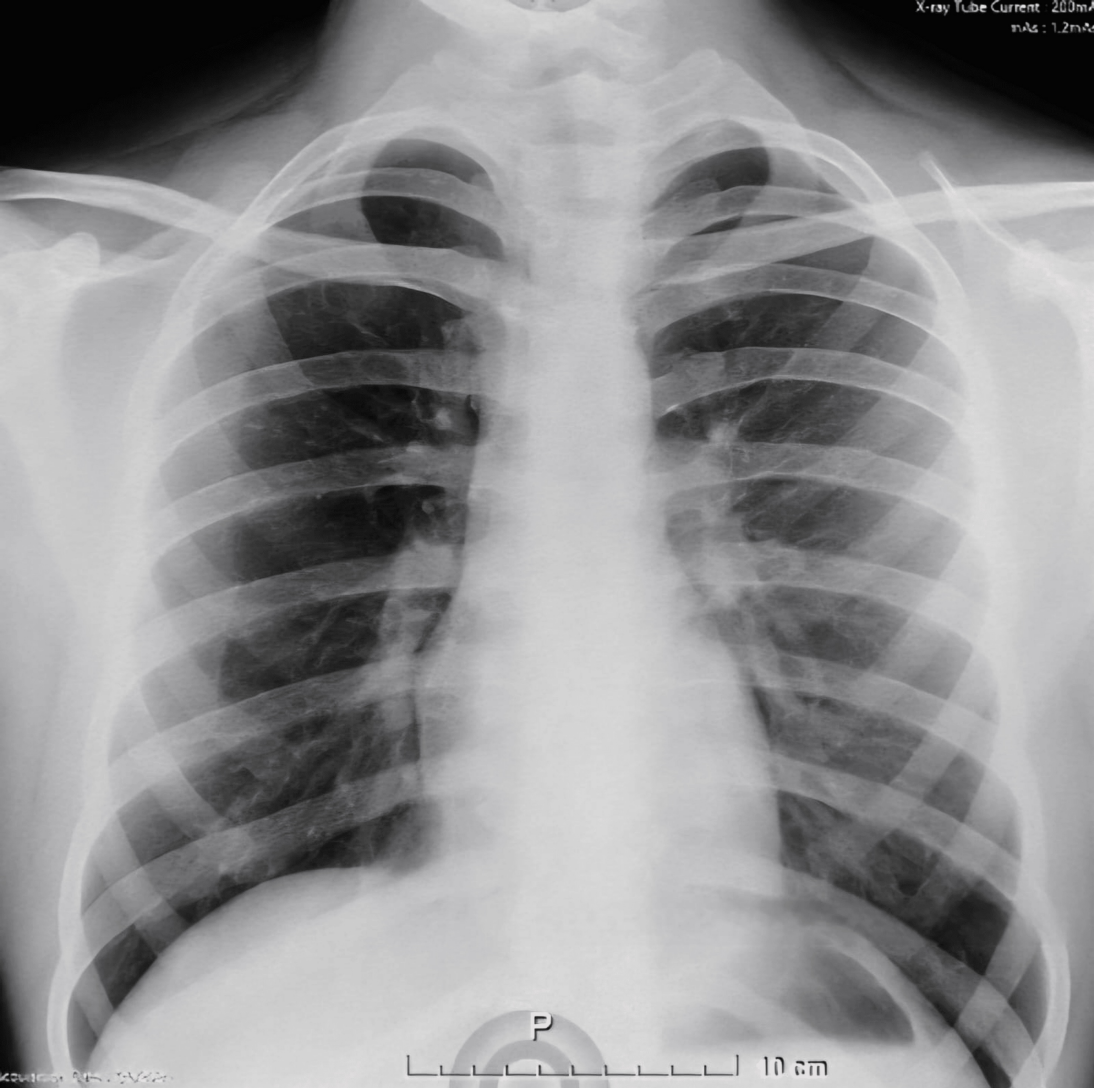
Chest X-ray The lungs are well aerated with no evidence of infiltrates, consolidations, masses, or pleural effusion. The mediastinum and vascular structures are within normal limits. The diaphragm has sharp contours with no elevation or flattening. Costophrenic and cardiophrenic angles are clear. Bony structures show no apparent abnormalities
